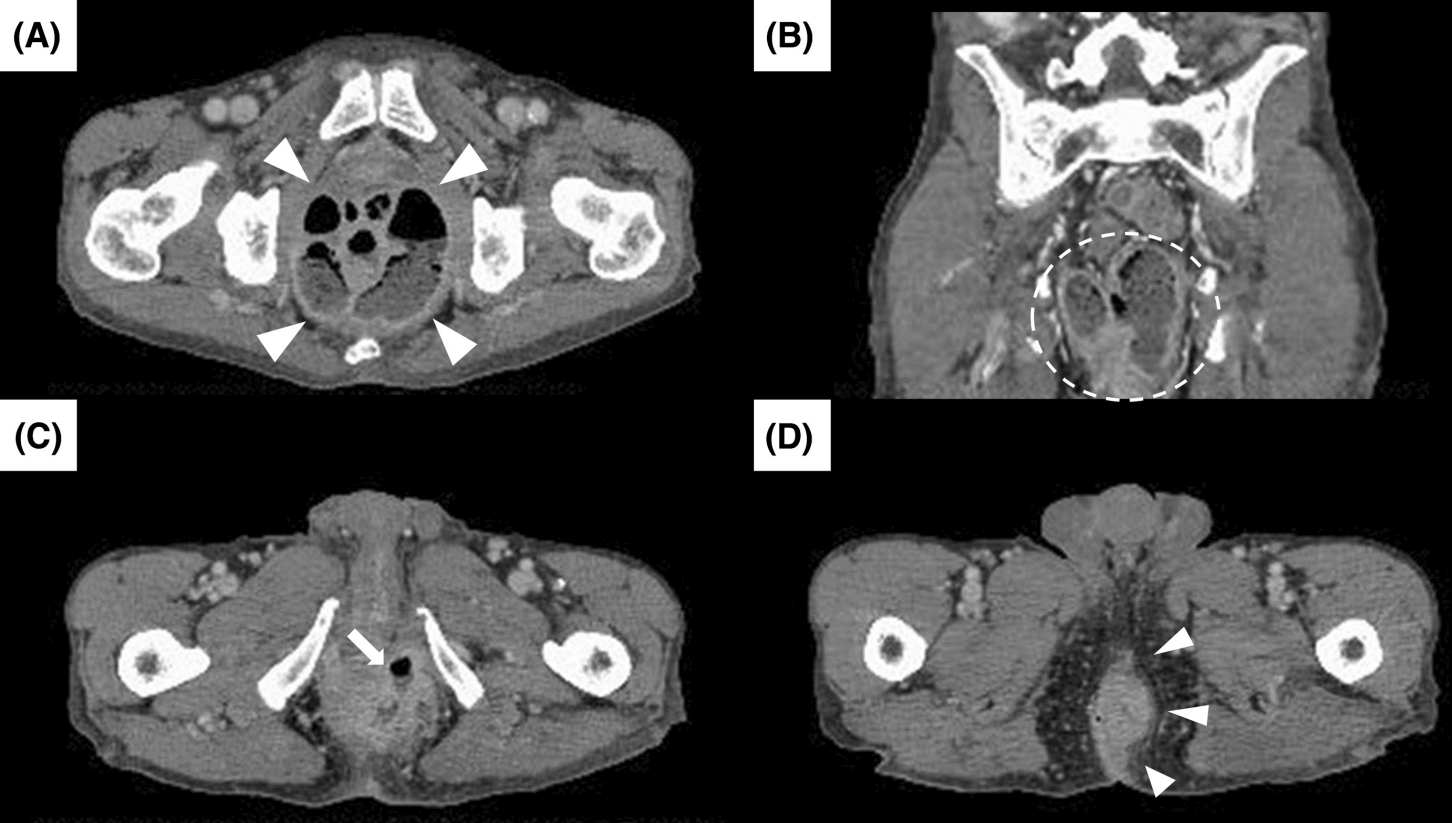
Thoracoabdominal contrast‐enhanced computed tomography (CT) images (A, C, D): horizontal sections; (B): coronal section. CT revealed a massive, encapsulated abscess with soft tissue shadows and air from the rectum to the perianal area ((A), arrowheads; (B), dotted circle, anal fistula at 2 o'clock (C), arrow), and swollen soft tissue on the left side of the anus ((D), arrowheads).
